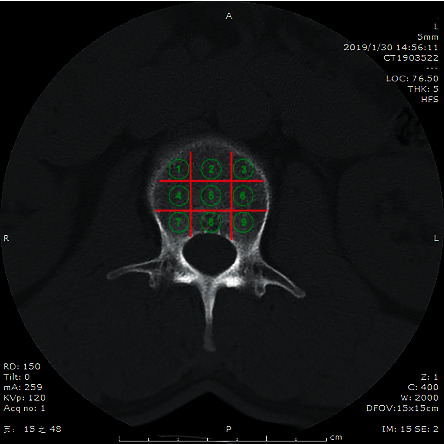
Regional CT values of the lumbar spine were measured by the nine-zone method.
